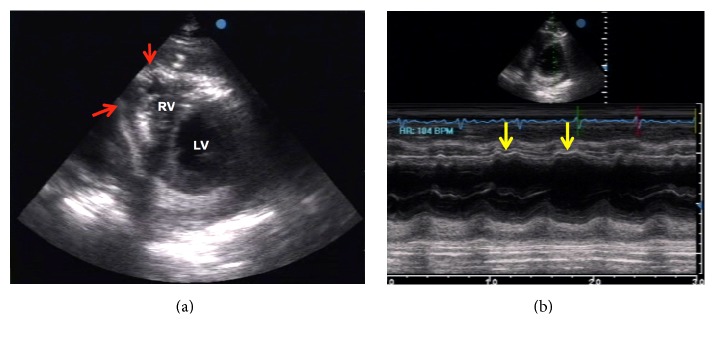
Echocardiographic parasternal short axis views at the level of the mid-left ventricle (LV). (a) The free wall of the right ventricle (RV) appears adherent to the adjacent pericardium (red arrows). There was note of septal bounce, leading to a D-shaped LV cavity during diastole. (b) M-mode of the same view confirmed the wavy motion (yellow arrows) of the interventricular septum representing septal bounce.
